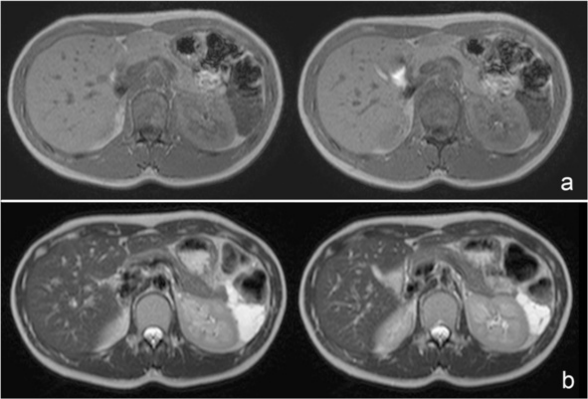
Magnetic resonance imaging revealed the mass low intensity in the T1-weighted image (a) and high intensity in the T2-weighted image (b)
